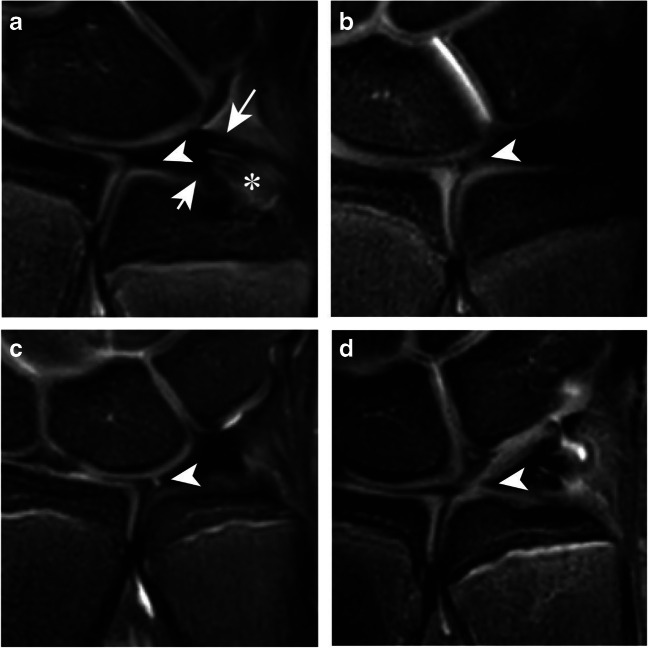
Coronal proton-density weighted fat-saturated MRI showing TFCs (indicated with arrowheads) with a hypo-intense signal intensity, b diffuse hyper-intense signal intensity not extending through the articular surface, c vertical linear hyper-intense signal intensity with discontinuation of the disc, and d a broader variation on this vertical line. Image a also shows the proximal (short arrow) and distal lamina (long arrow) as homogeneous hypo-intense bands, separated by the ligamentum subcruentum shown as a hyper-intense signal intensity (asterisk)
